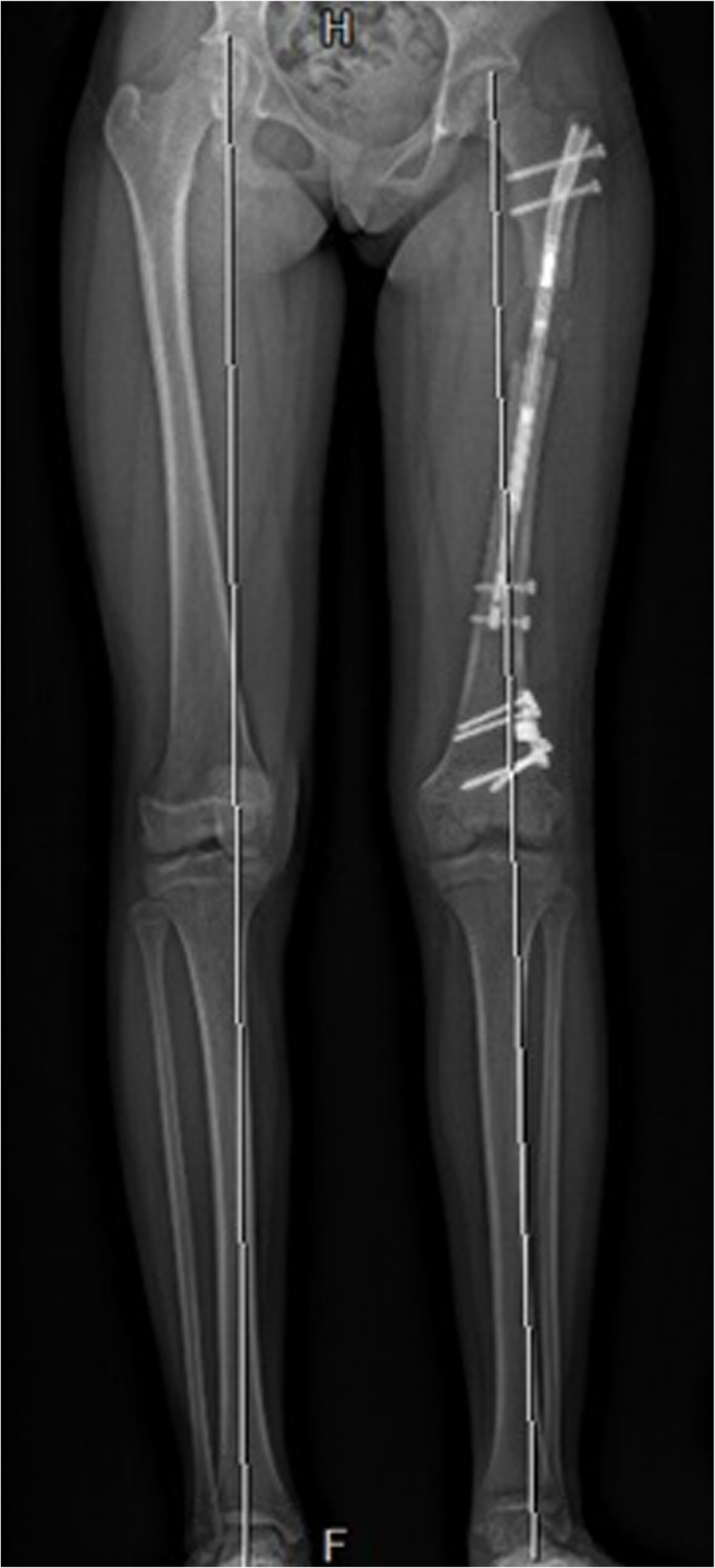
X-ray at a follow-up visit demonstrates interval lengthening and good alignment of the weight-bearing axis
